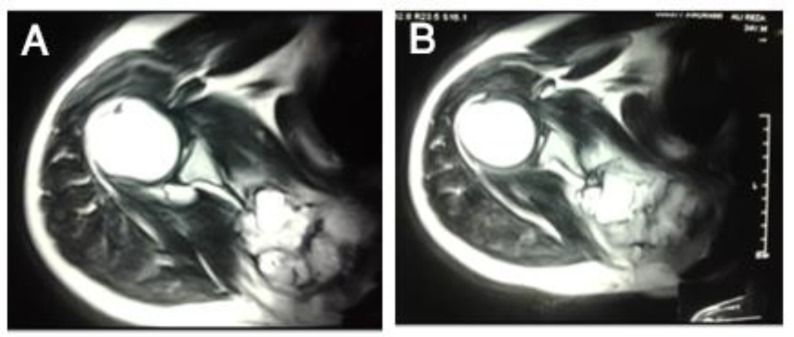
Magnetic Resonance Imaging of right shoulder at recurrence showing a large expansile hypersignal mass in medial aspect of supraspinatus muscle, within the body of scapula below the level of clavicle
